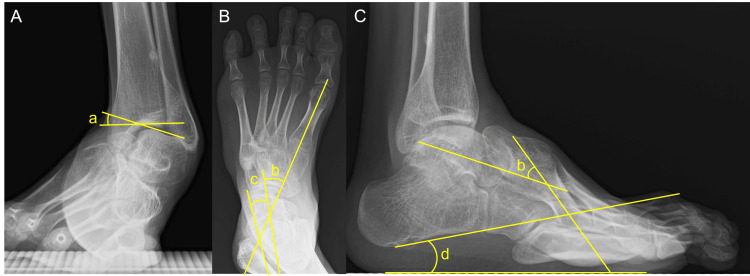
Preoperative weight-bearing radiographs (A) Anteroposterior view of the ankle; (B) anteroposterior view of the foot; (C) lateral view of the foot. (a) Talar tilt angle; (b) talo-first metatarsal angle; (c) talo-calcaneal angle; (d) calcaneal pitch angle
